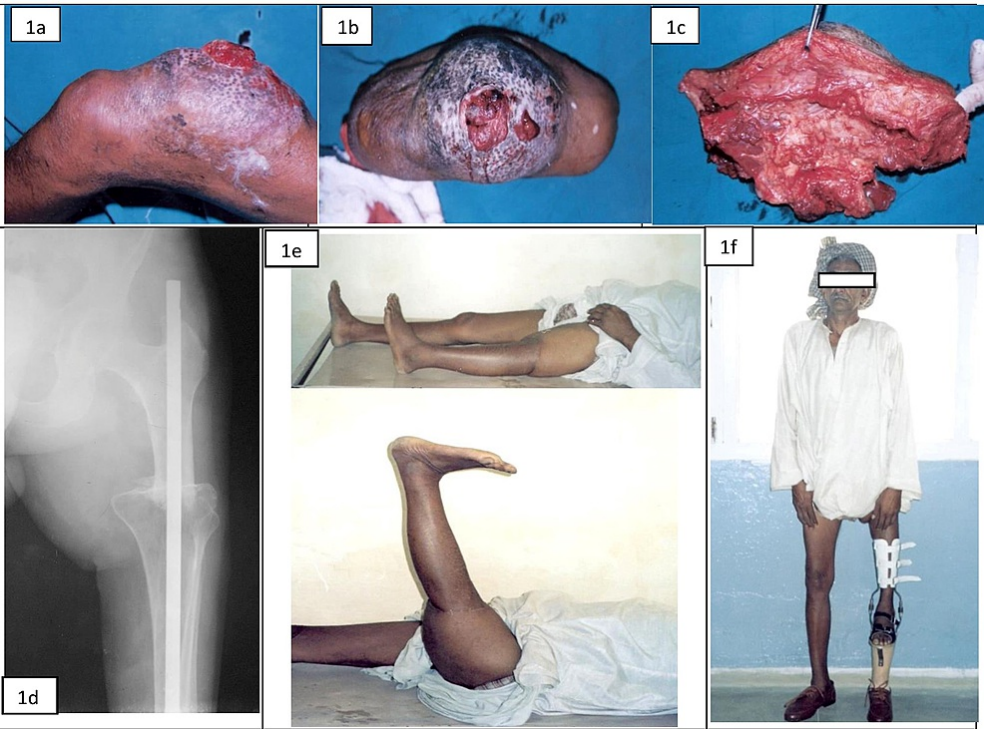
A 45-year-old male with a diagnosis of malignant fibrous histiocytoma (MPH) distal thigh (First row) 1a (side view) and 1b (front view), showing clinical pictures of angiomatoid type variant; 1c, excised tumoral mass with soft tissue components; (second row); 1d, radiographs showing well-healed osteosynthesis of the lower limb with intramedullary nailing; 1e, clinical picture with full functional shortened straight limb; 1f, showing the patient standing with the custom-made extension prosthesis.
